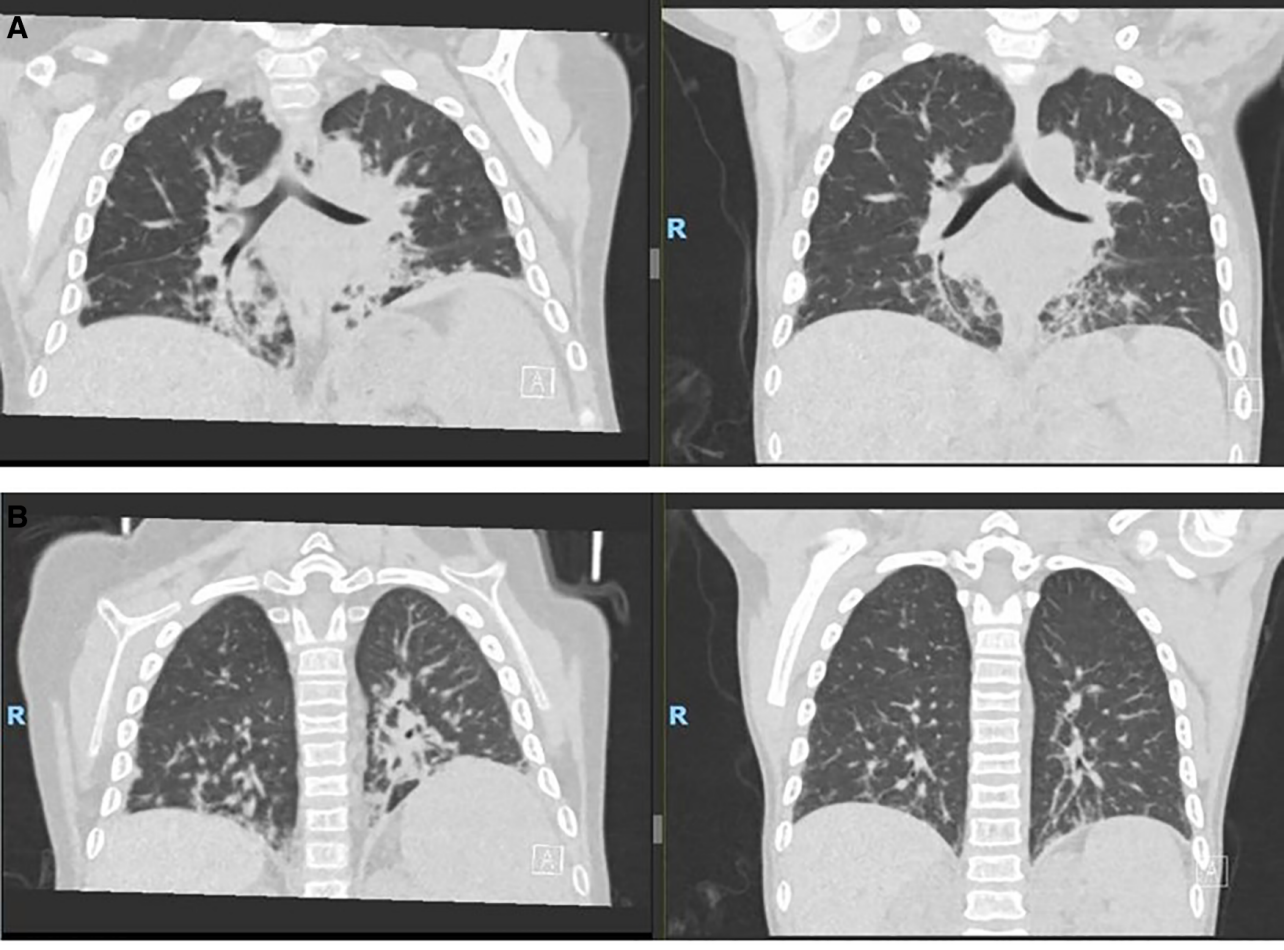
Ct scans. (A) Pre–allo-HSCT. (B) 9 months post–allo-HSCT. CT, computed tomography; allo-HSCT, allogeneic hematopoietic stem cell transplantation.
